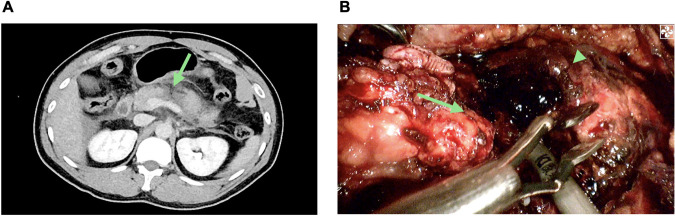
Isolated pancreatic trauma. (A) Abdominal computed tomography (CT) showing discontinuity at the junction site of the pancreas neck and body (the green arrow). (B) Pancreatic disconnection seen on laparoscopic exploration; proximal pancreatic remnant (the green arrow); distal pancreatic remnant (the green arrow head).
